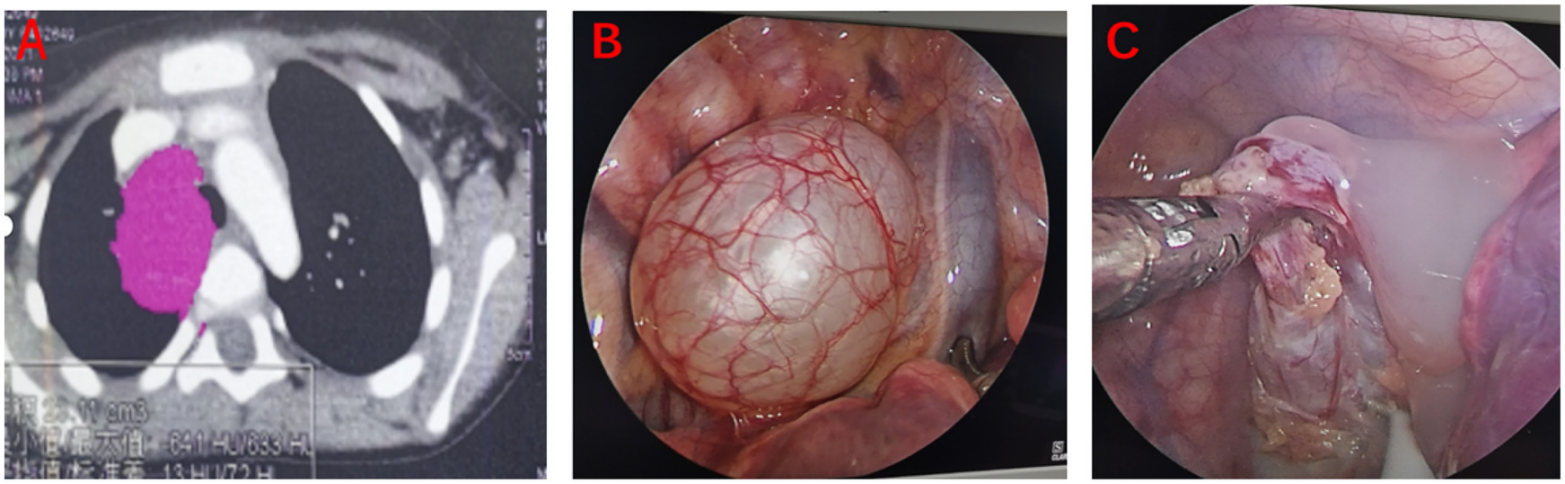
Bronchogenic cyst. (A) Preoperative CT scan; (B) Intraoperative view of the intact cyst; (C) Cystic fluid after decompression.
